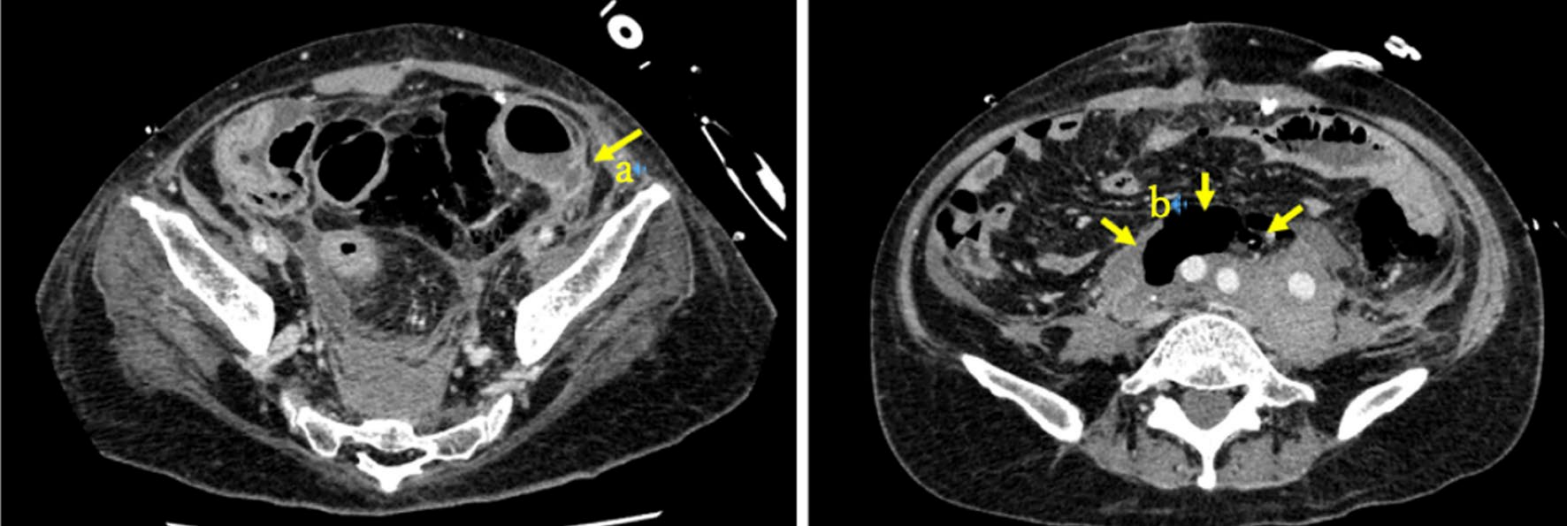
Computed tomography of the abdominal to pelvic region on postoperative day 11 shows a tear of the intestinal wall dorsal to the sigmoid colon, with a suspected perforation at the same area (arrow a). Free air is seen late in the retroperitoneum in front of the graft (arrow b)
